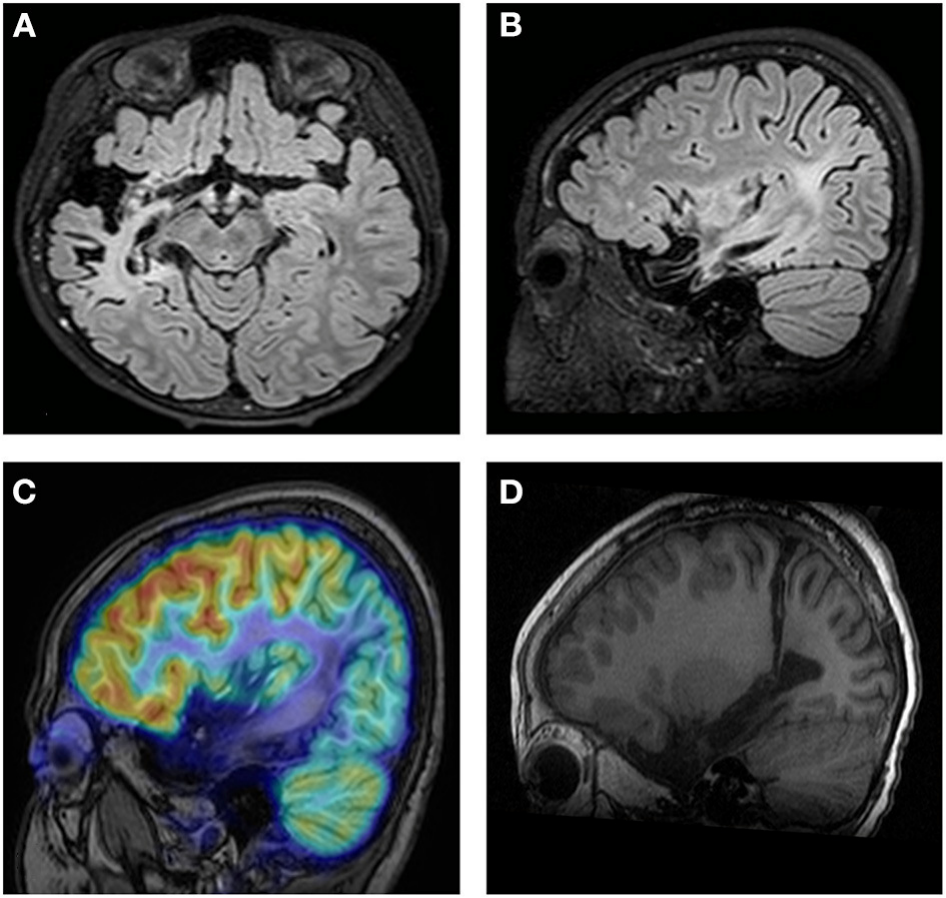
Neuroimaging data of case 1. MRI reveals encephalomalacia in the right temporal lobe (A). Abnormal signals are observed in the right parietal and occipital lobes (B). PET-MRI Coregistration shows hypometabolism in the temporal, parietal and occipital lobes (C). Postoperative MRI shows disconnection of the temporo-parieto-occipital lobes (D).
